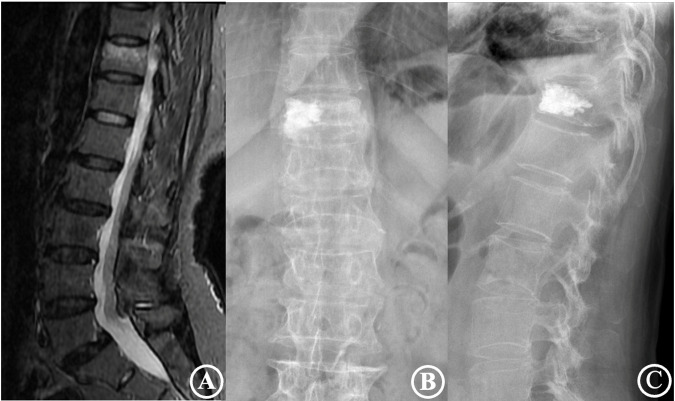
The patient was a 75-year-old male diagnosed with an osteoporotic vertebral compression fracture of T11. (A) is a preoperative MRI film suggesting a fresh vertebral compression fracture of the T11 vertebra; (B,C) are frontal and lateral X-rays after percutaneous vertebroplasty, showing a type IV cement distribution and poor cement dispersion.
